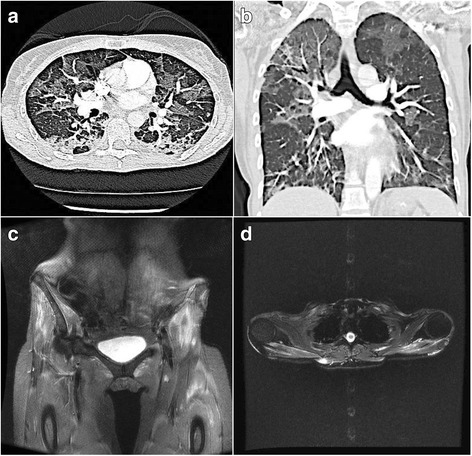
Axial (a) and Coronal (b) CT (lung windows). Multifocal ground glass opacity progressing to consolidation in the dependent lungs. (d) Coronal STIR Pelvis: T2 hyperintensity in gluteal, quadriceps and adductor musculature in keeping with myositis. (e) Axial T2FS Upper limbs: Further oedema within trapezius, deltoid and rotator cuff musculature
